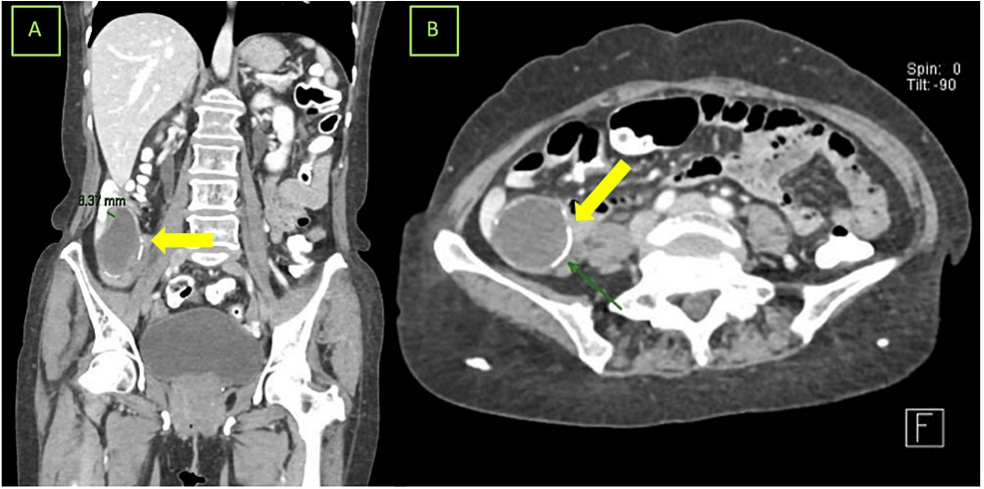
Computed tomography scan (A) Coronal section: The yellow arrow shows a homogeneous cystic-appearing lesion with regular borders and irregular calcification of the walls at the appendicular base, extending to the cecum and terminal ileum without distant activity. (B) Axial section: A mucinous cystic neoplasm at the appendicular base measuring 13.2 x 6.4 x 4.4 cm with regular borders and calcified walls, without evidence of invasion into mesocolonic lymph nodes is seen.
